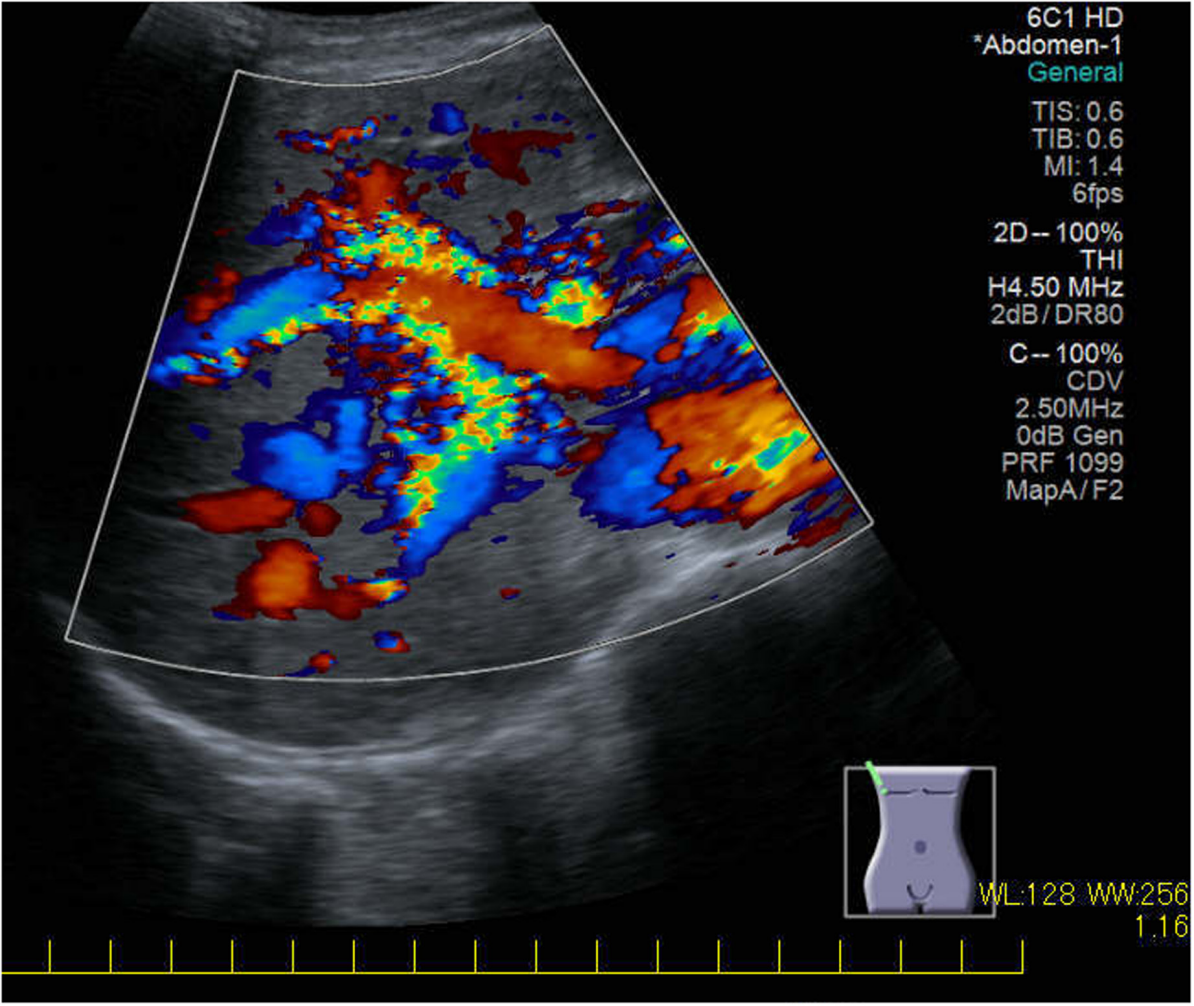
Abdominal ultrasonography revealed that the intrahepatic portal vein was prominent and increased blood flow.
